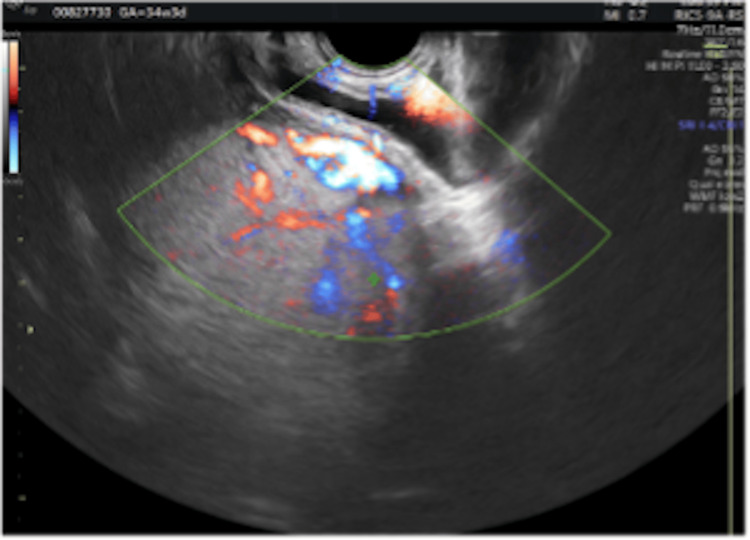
Ultrasound showing placenta accreta (Case 2).
